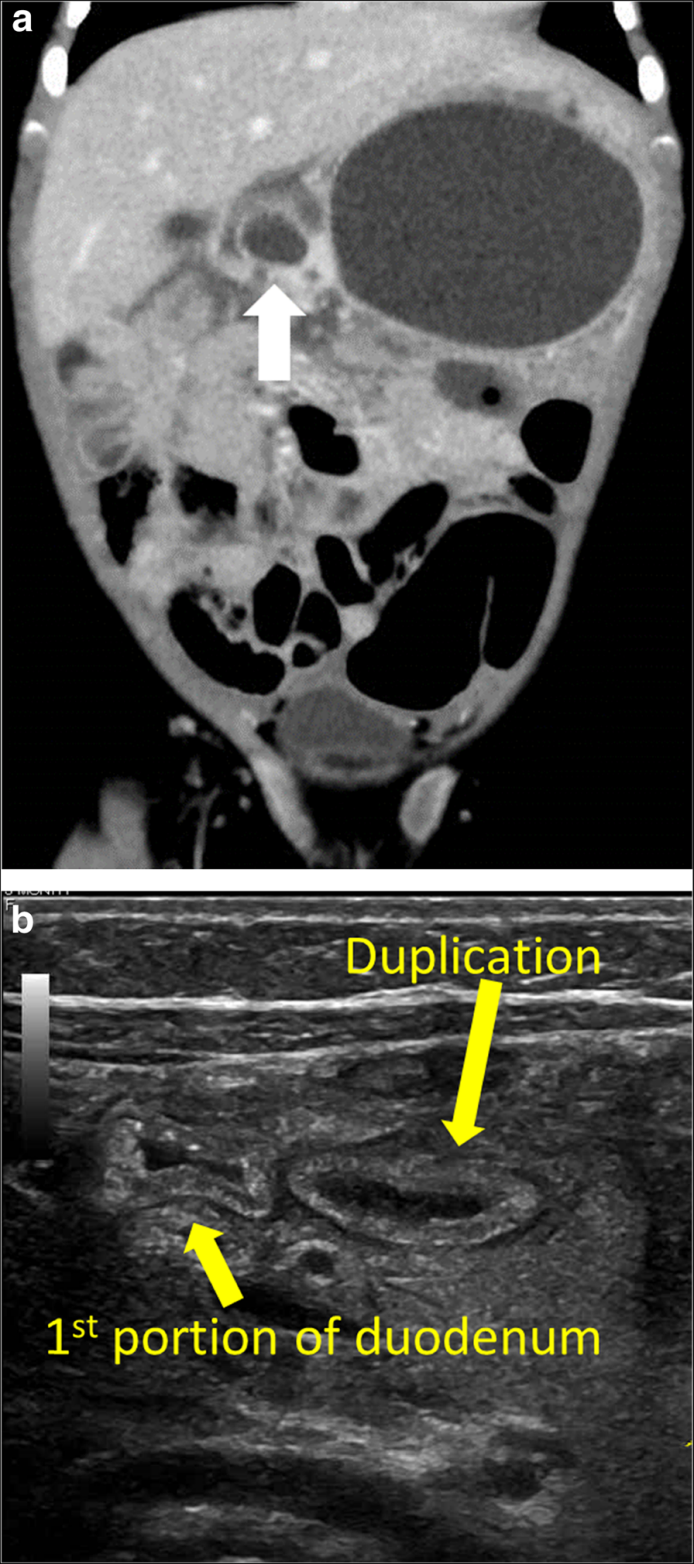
Post-surgical imaging, case 1. a Computed tomography (CT) reveals a large pseudopancreatic cyst and a duodenal duplication cyst (white arrow) attached to the first portion of the native duodenum. b Ultrasonography (sagittal view) shows duplication at the first portion of the duodenum with hyperechoic mucosa surrounded by hypoechoic muscular layers
